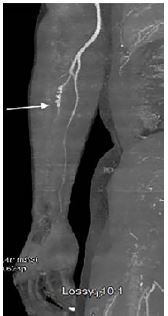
Computed tomographic angiography of the right upper extremity revealing abrupt non-opacification of the ulnar artery approximately three centimeters below the takeoff of the interosseous artery (arrow). Non-visualization of the superficial palmar arch and metacarpal arteries.
